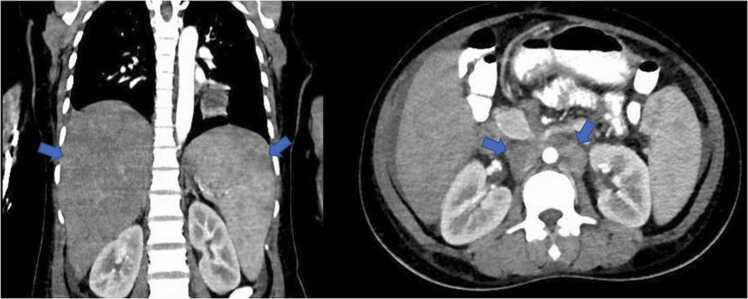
CT abdomen showing hepatosplenomegaly (left),and bulky enlargement of para-aortic lymph nodes (right).
